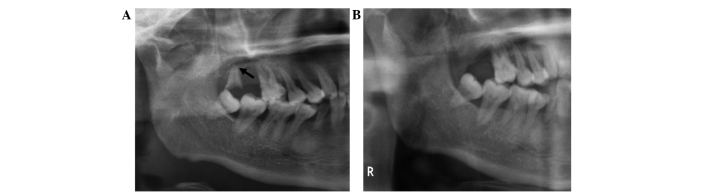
(A) Preoperative panoramic radiography shows the periapical lesions of the right maxillary third molar residual root (shown by the black arrow). (B) Postoperative panoramic radiography demonstrates the extraction of the residual root.
